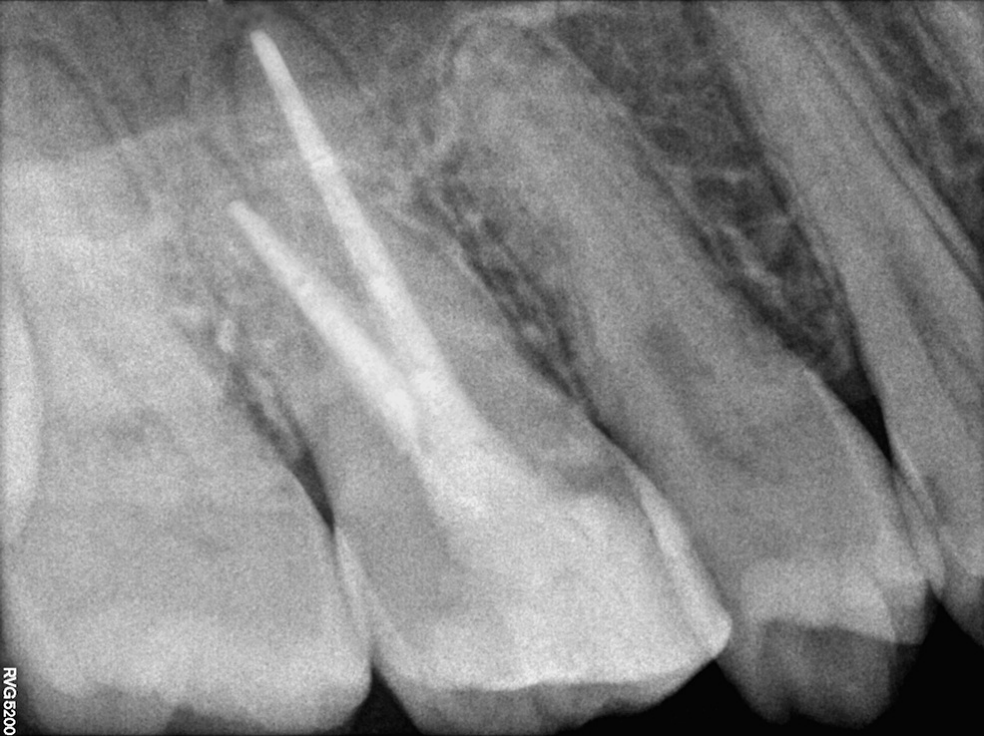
Post-obturation radiograph.
